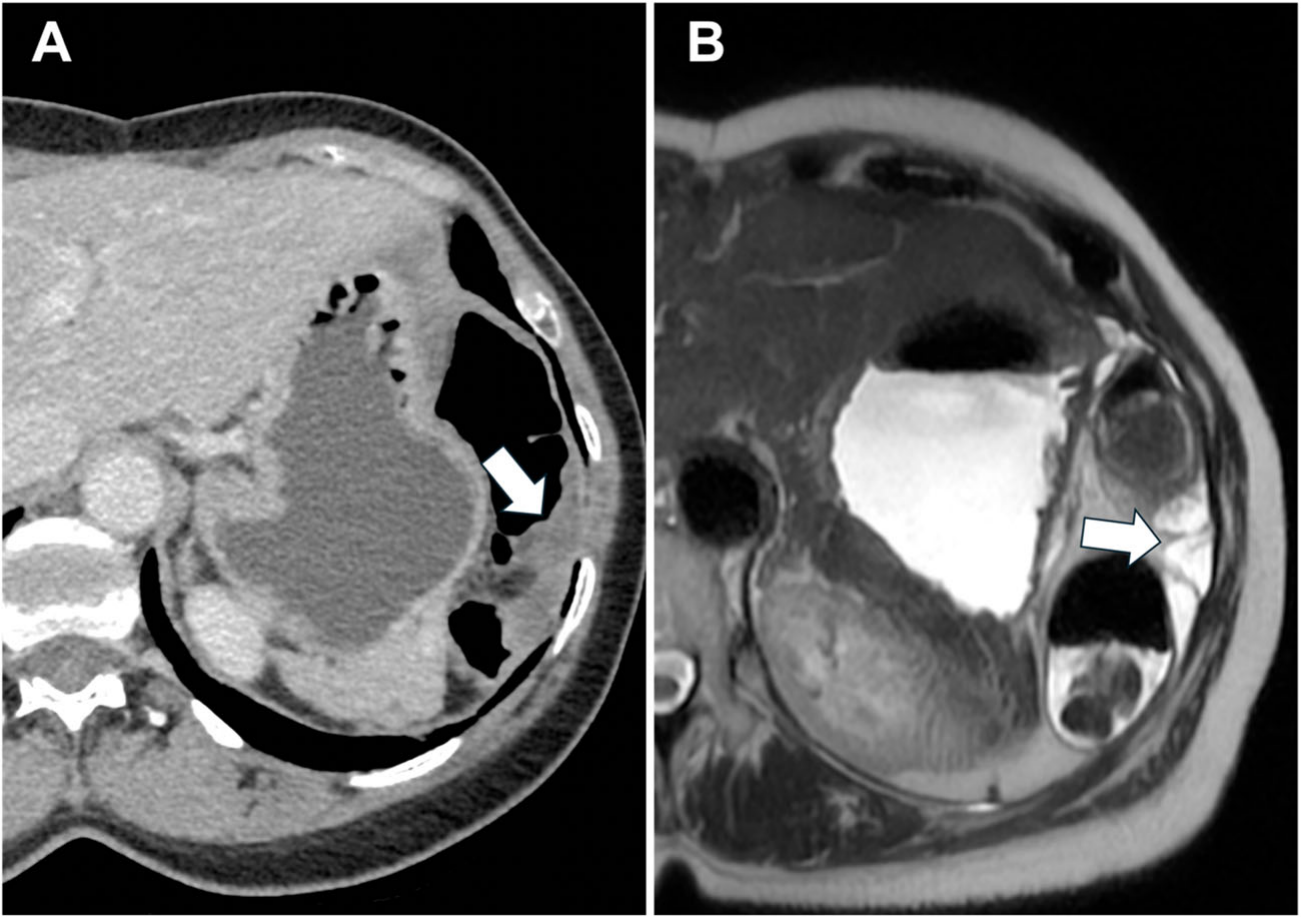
Female, 56‐year old, affected by peritoneal mesothelioma with loculated ascites on computed tomography portal venous phase (A) and magnetic resonance imaging T2w (B) (white arrows).
